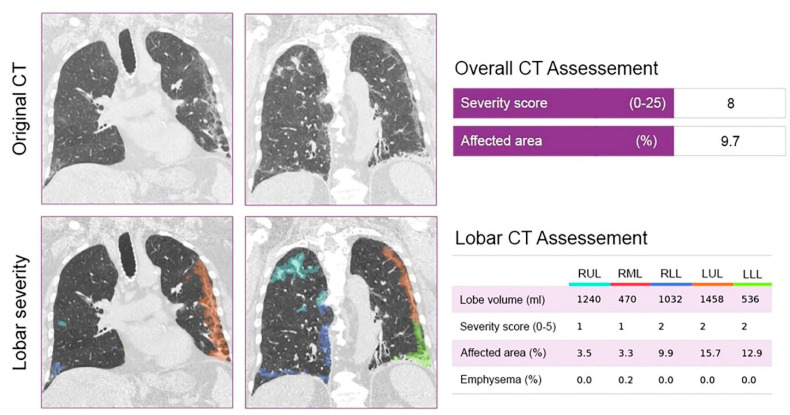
Representative example of the AI-based CAD4COVID–CT software of a patient with a total CT severity score of 8. The original and AI–assessed chest–CT of a 67–year old male patient, who was hospitalized with an SpO2 of 95% at the time of hospital admission. The patient was receiving chemotherapy for prostate cancer at the time of the CT scan. As a result of the standard therapy, the patient experienced gradual improvement in his condition during hospitalization and was released home after 10 days. CT severity scores, affected areas, lobe volumes and emphysema areas are reported on the right side. Severity scores were calculated using the percentage of the affected area of the parenchyma. Abbreviations: CT = computed tomography.
